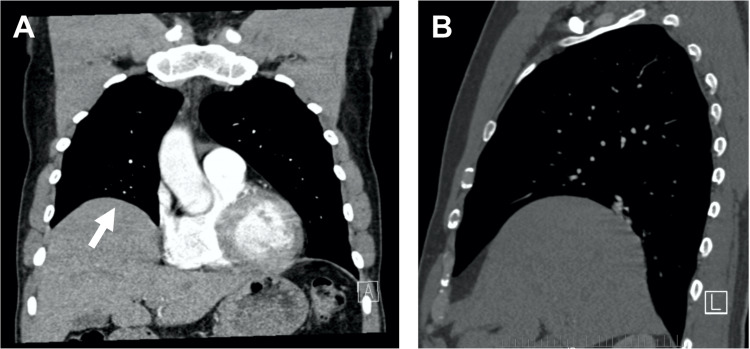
Eventration of the right hemidiaphragm CT scan of the chest demonstrating eventration of the right hemidiaphragm. The white arrow in the coronal view (Panel A) indicates the area of diaphragmatic eventration. Panel B shows a sagittal view of the diaphragmatic eventration.
